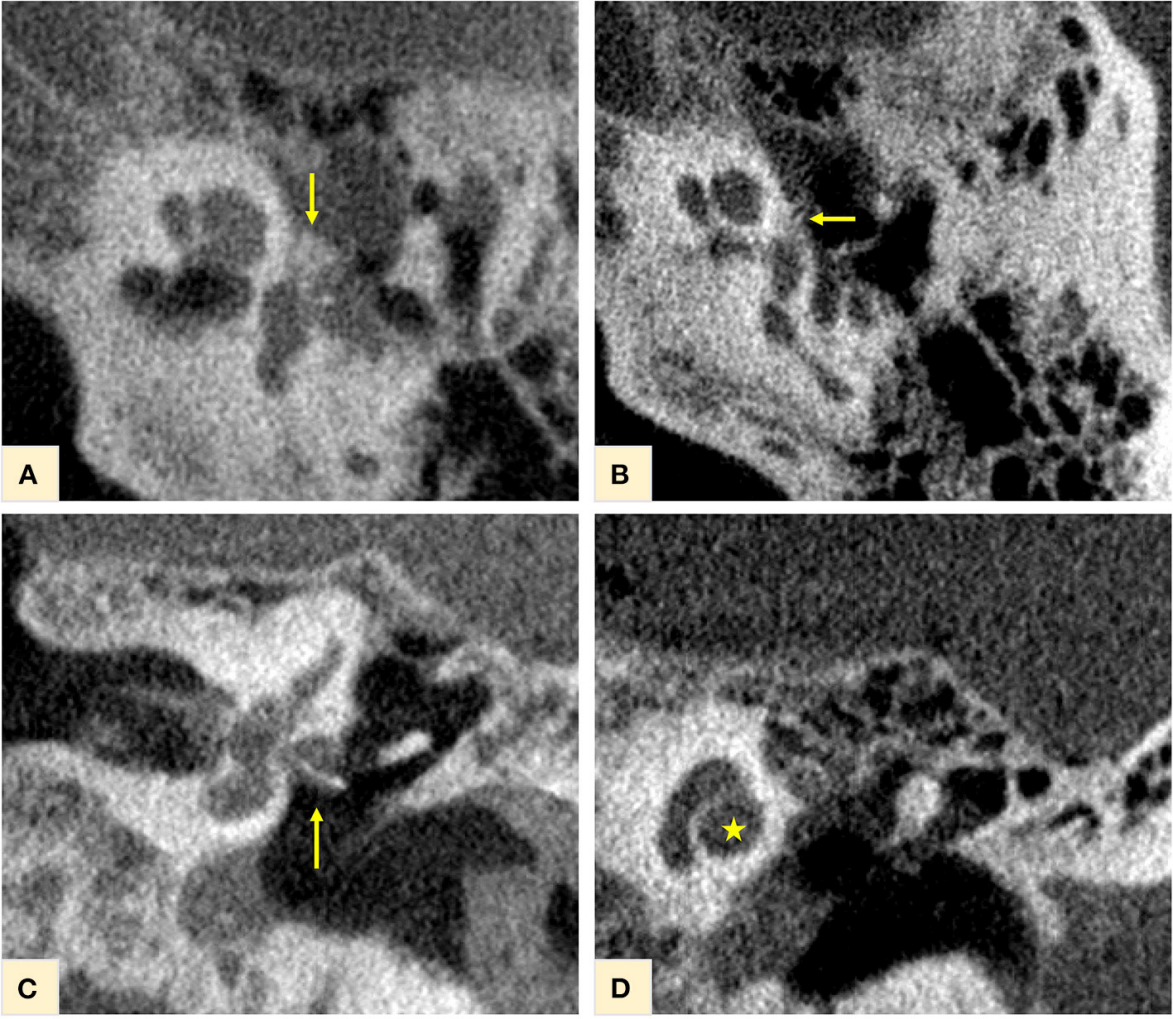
CBCT of the temporal bone. Otospongiotic plaques in *loco typico* confirming the disease diagnosis, were clearly recognizable at the fissula ante fenestrum, with involvement of the oval window and the “otosclerotic angle” [yellow arrows in (A,B)]. The titanium stapes prosthesis *in situ* for disease treatment is shown on (C) (yellow arrow). At this initial stage of the disease, no other otospongiotic foci were seen and the cochlea appeared with a normal contour on (D) (yellow star).
